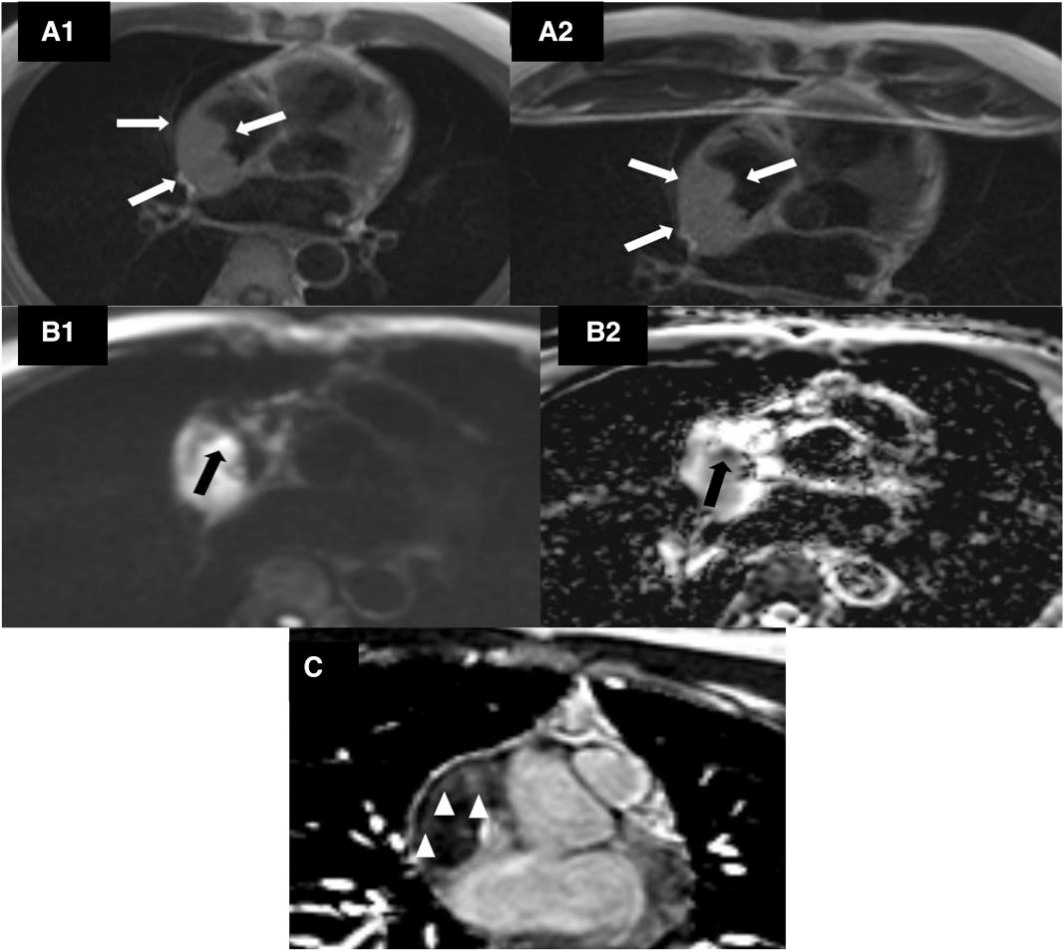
(*A1* and *A2*) Cardiac magnetic resonance imaging, T1 and T2-weighted TSE sequences, the mass is homogeneous and slightly hyperintense compared to the muscle (white arrows). (*B1* and *B2*) In the diffusion and ADC map with trace b = 1000 s/mm^2^, it is seen that some regions within the mass are markedly diffusion restricted (black arrow). The lowest ADC value was ∼550 × 10 −6 mm²/s. (*C*) Transverse plane ‘phase-sensitive inversion recovery’ sequence showing mild late gadolinium enhancement in the mass (arrowheads).
